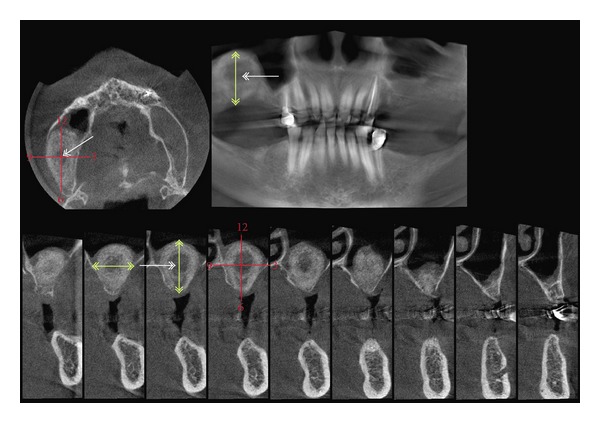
CBCT scan measurements: yellow arrows: total distances height/width/depth, red reference cross: measurements of calcification thicknesses in 3, 6, 9, and 12 o'clock position.
